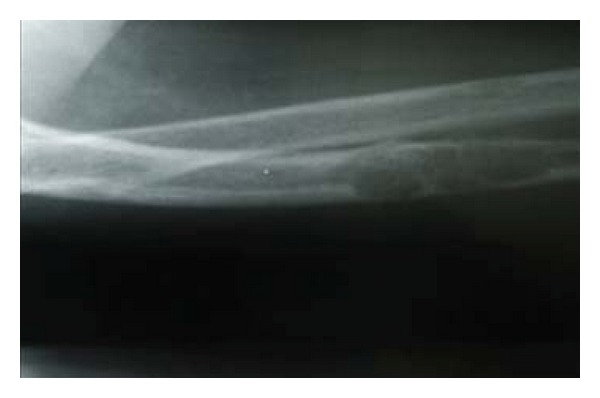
Lateral X-ray of the right radioulna showing osteitis fibrosa cystica lesion of ulna diaphysis with a pathologic fracture (before curettage).
